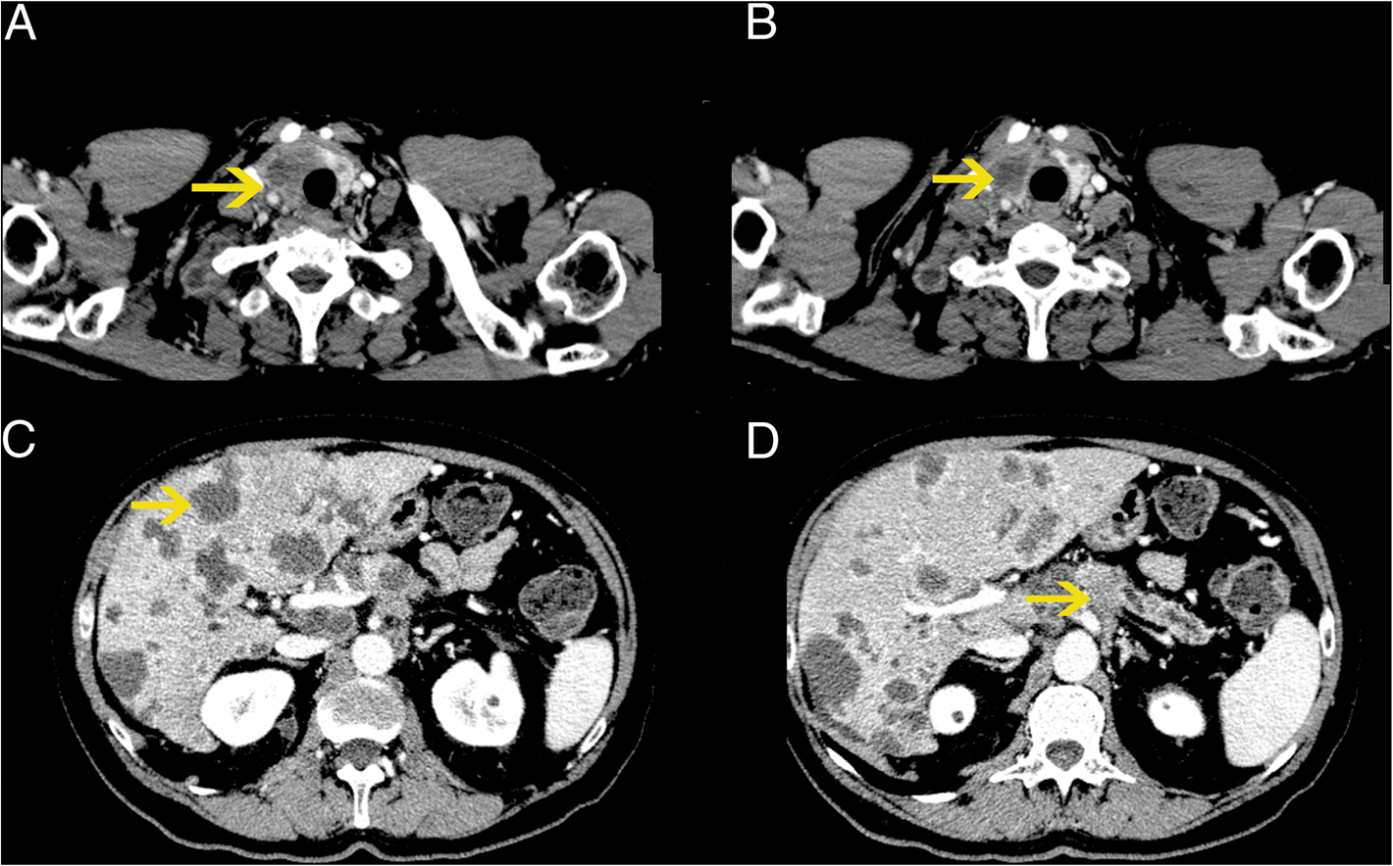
CT scan of the body. In panel A and B the yellow arrows indicate the big mass of the right lobe of the thyroid gland. In panel C the yellow arrow indicates one of the multiple secondary liver lesions. In panel D the yellow arrow indicates the pancreatic cancer located in the body with dilatation of the pancreatic duct.
